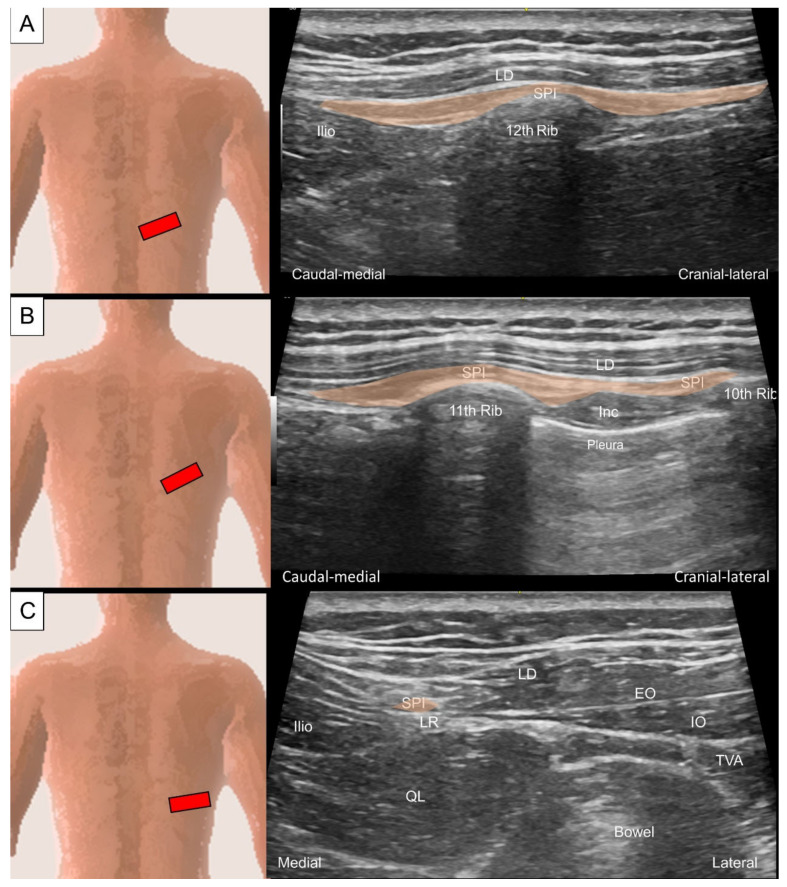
Ultrasound imaging of the serratus posterior inferior (SPI) at the level of (A) the 12th rib, (B) between the 11th and 10th ribs, and (C) beside the lateral raphe (LR) where the quadratus lumborum (QL), paraspinal muscles, and abdominal muscles merge. LD, latissimus dorsi; Ilio, iliocostalis; Inc, intercostal muscle; EO, external oblique muscle; IO, internal oblique muscle; TVA, transversus abdominis.
